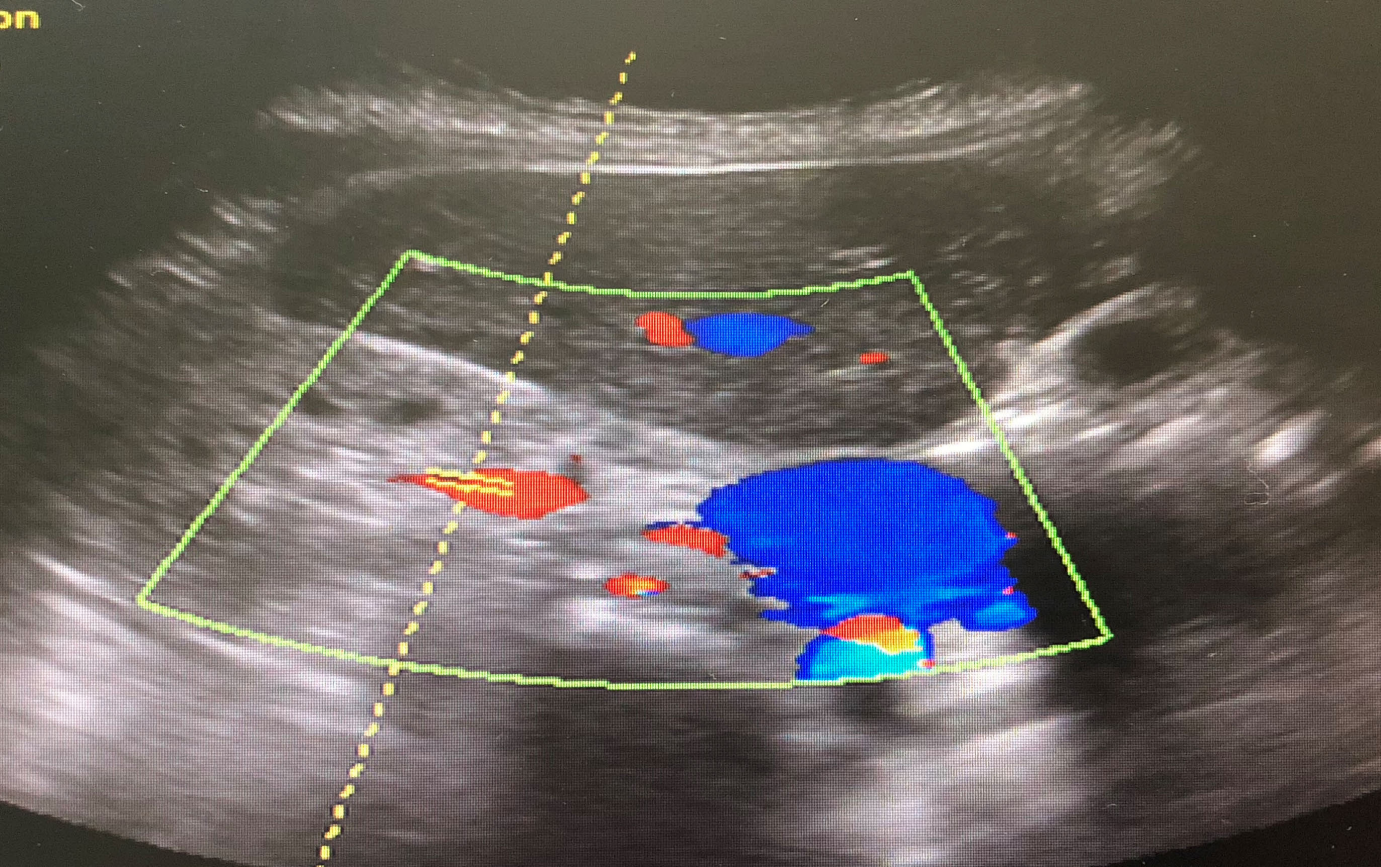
Renal Doppler ultrasound: abnormal resistive index bilaterally with abnormally echogenic kidney, suggestive of medical renal disease
